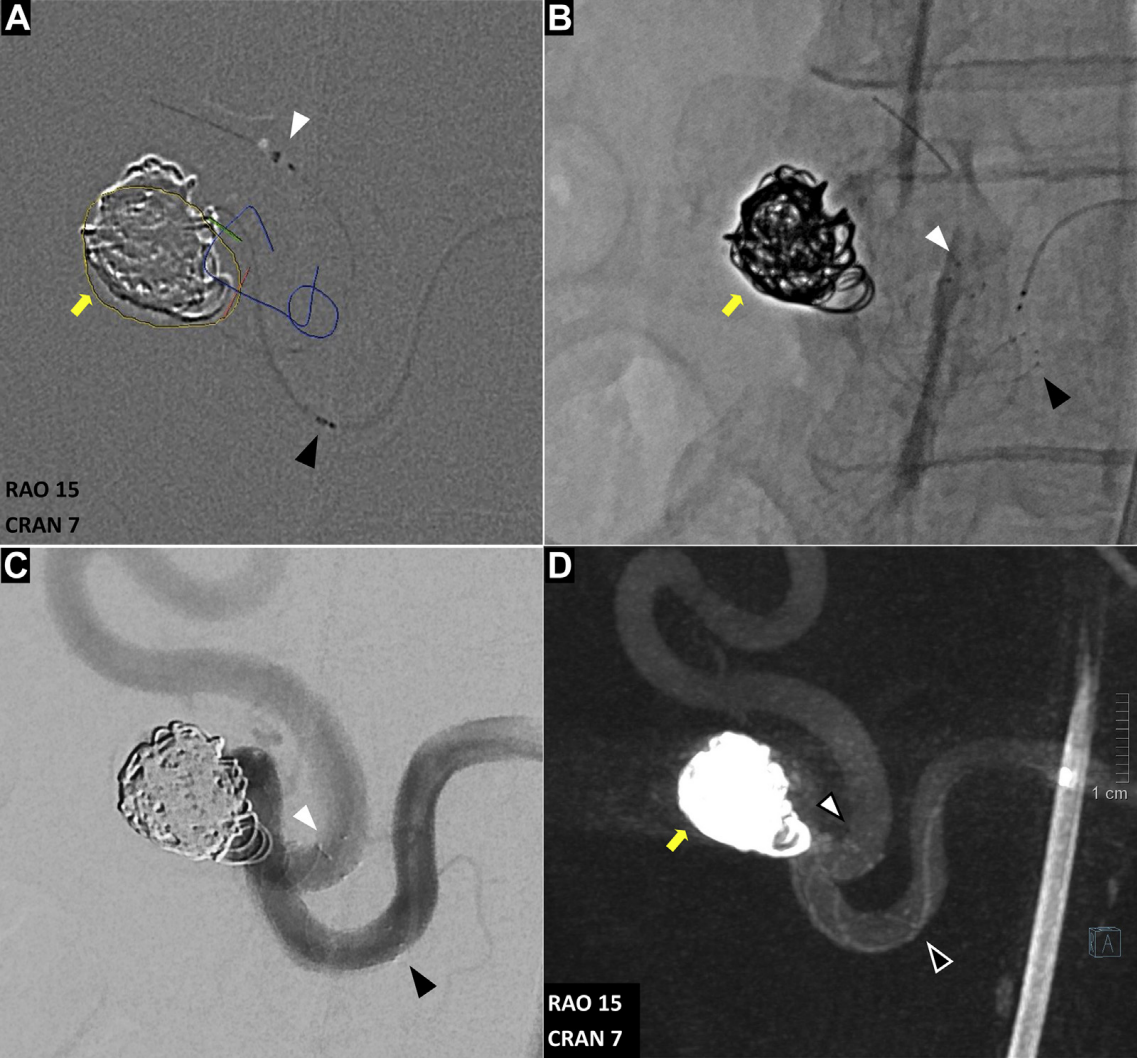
A, Deployment of low-profile visualized intraluminal support (LVIS) flow-diverter stent with two-dimensional (2D) road mapping and cone-beam computed tomography angiography (CBCTA) image fusion guidance. Planned proximal and distal landing zones were electronically marked on the CBCTA images (*blue rings* and *blue centerline*), overlaid on 2D fluoroscopy and roadmap images. B, The proximal (*black arrowhead*) and distal (*white arrowhead*) markers of the flow-diverting stent were positioned using information from CBCTA and deployed. Completion 2D angiography (C) and CBCTA (D) images demonstrating near complete occlusion of aneurysm sac with coil embolization and well-apposed flow-diverting stent in proximal (*black arrowhead*) and distal (*white arrowhead*) parent vessels.
